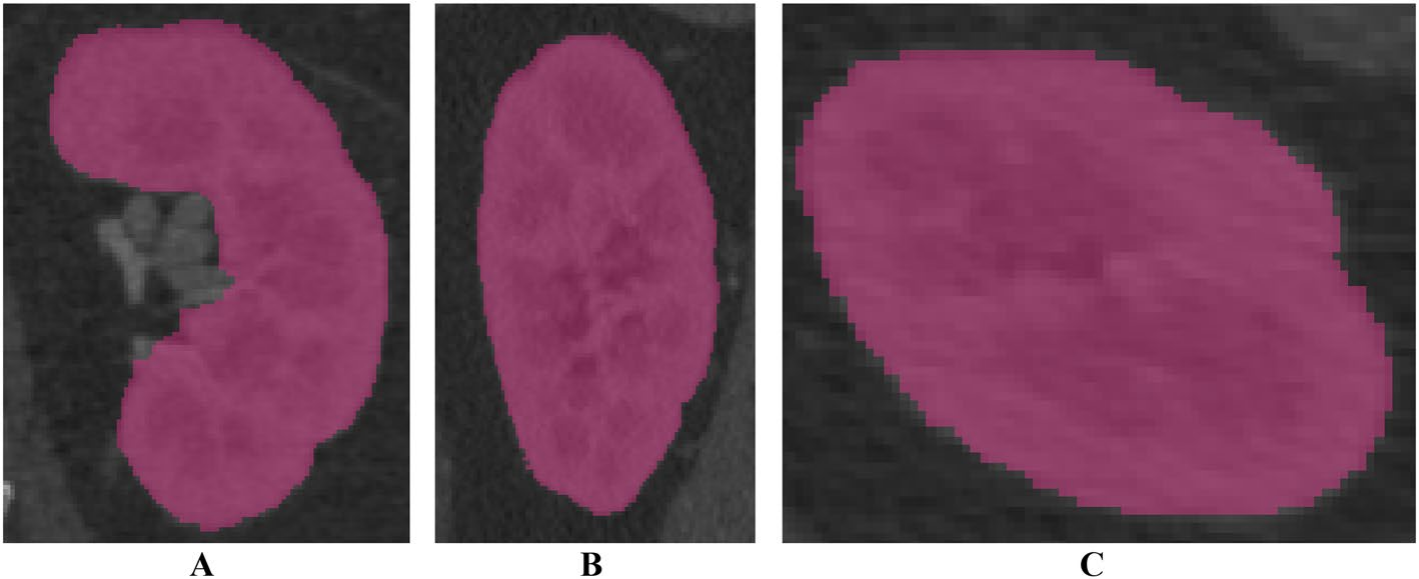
Visualization of the segmented left kidney in computed tomography images of a 47-year-old patient who was diagnosed with partial nephrectomy. The view of (A) coronal, (B) sagittal, and (C) axial.
